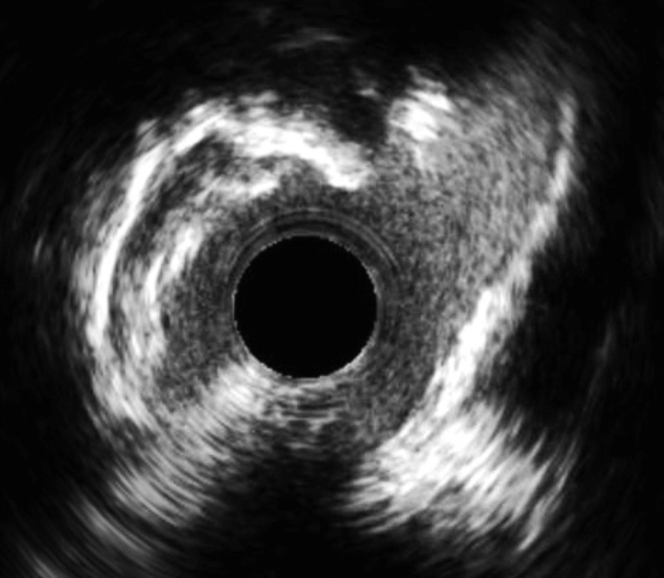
Intravascular Ultrasound of Left Main Stem Ostium
